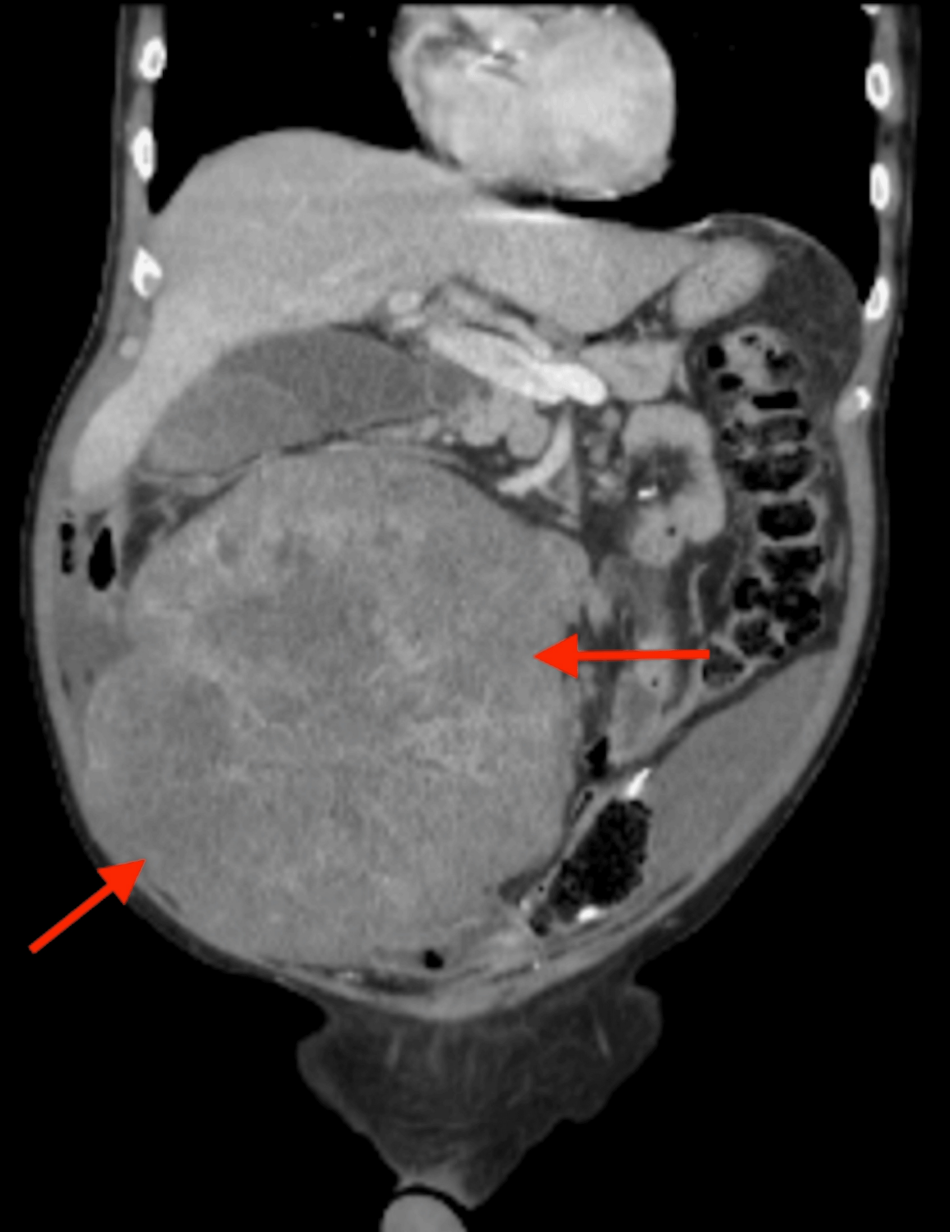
Coronal CT view of the abdomen and pelvis, showing the large complex soft tissue mass (red arrows) extending from the right upper quadrant into the lower abdomen CT: computed tomography
